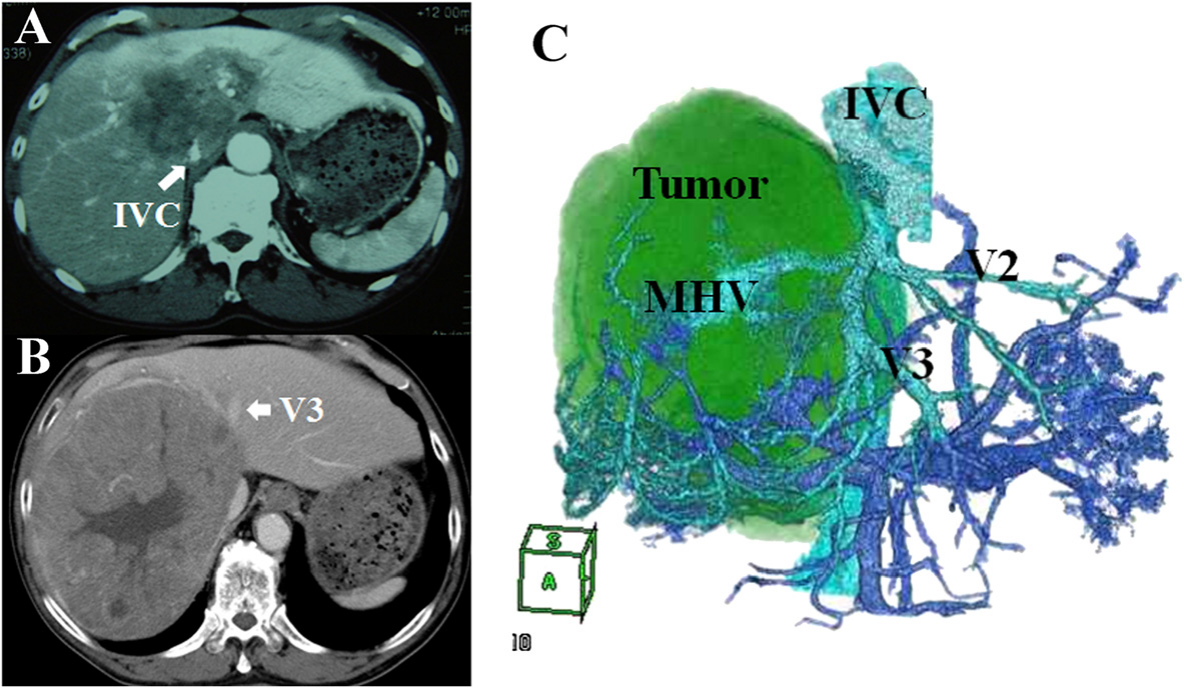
Preoperative enhanced computed tomography and reconstructed three-dimensional computed tomography. (A) Intrahepatic cholangiocarcinoma: tumor invasion of the inferior vena cava was suspected (patient 2). (B) Hepatocellular carcinoma: tumor invasion of the vein (V3) was suspected (patient 4). (C) Hepatocellular carcinoma: the left hepatic vein diverged to confluent V2 and V3 near the root and V3 was compressed by the tumor over a 3 cm length (patient 4). IVC, inferior vena cava; MHV, middle hepatic vein.
